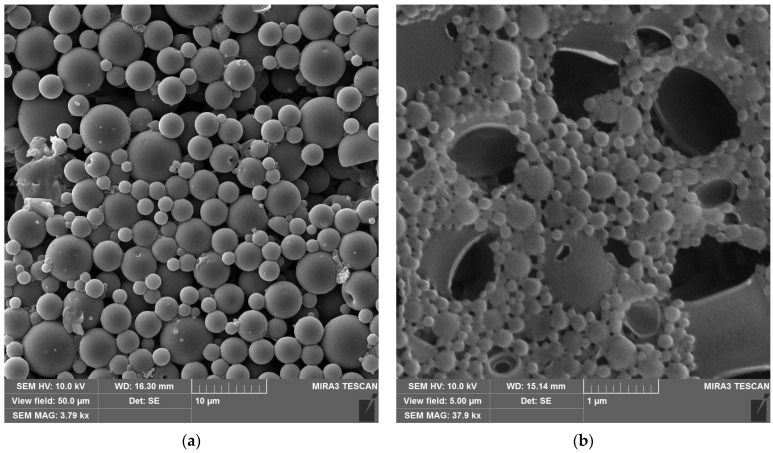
PLA suspension particles: (a) formed with mechanical homogenization; (b) formed with a combination of ultrasonic and mechanical homogenization.
